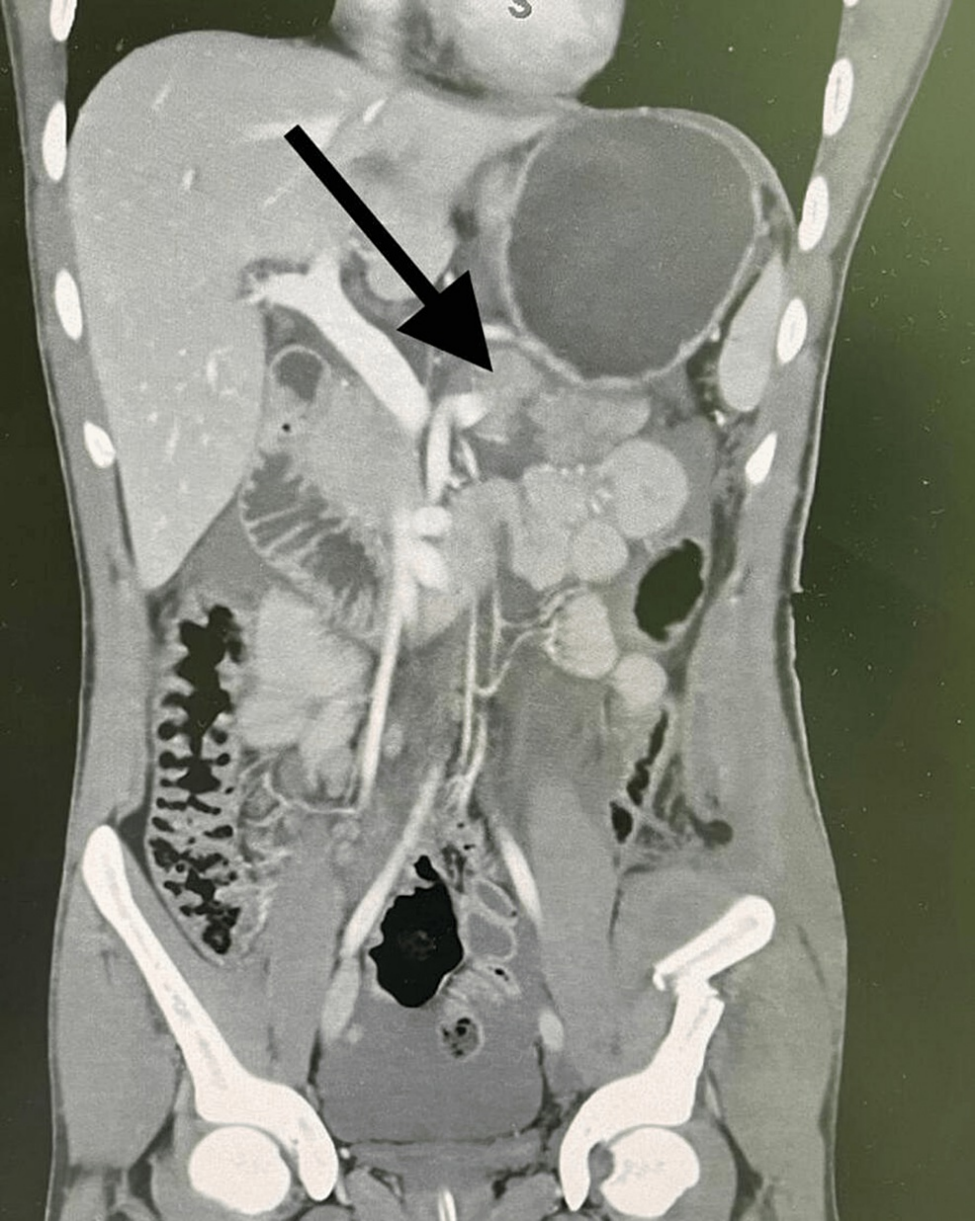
CT scan with contrast of the abdomen and pelvis, coronal view showed laceration through the body of the pancreas and peripancreatic fluid collection.
